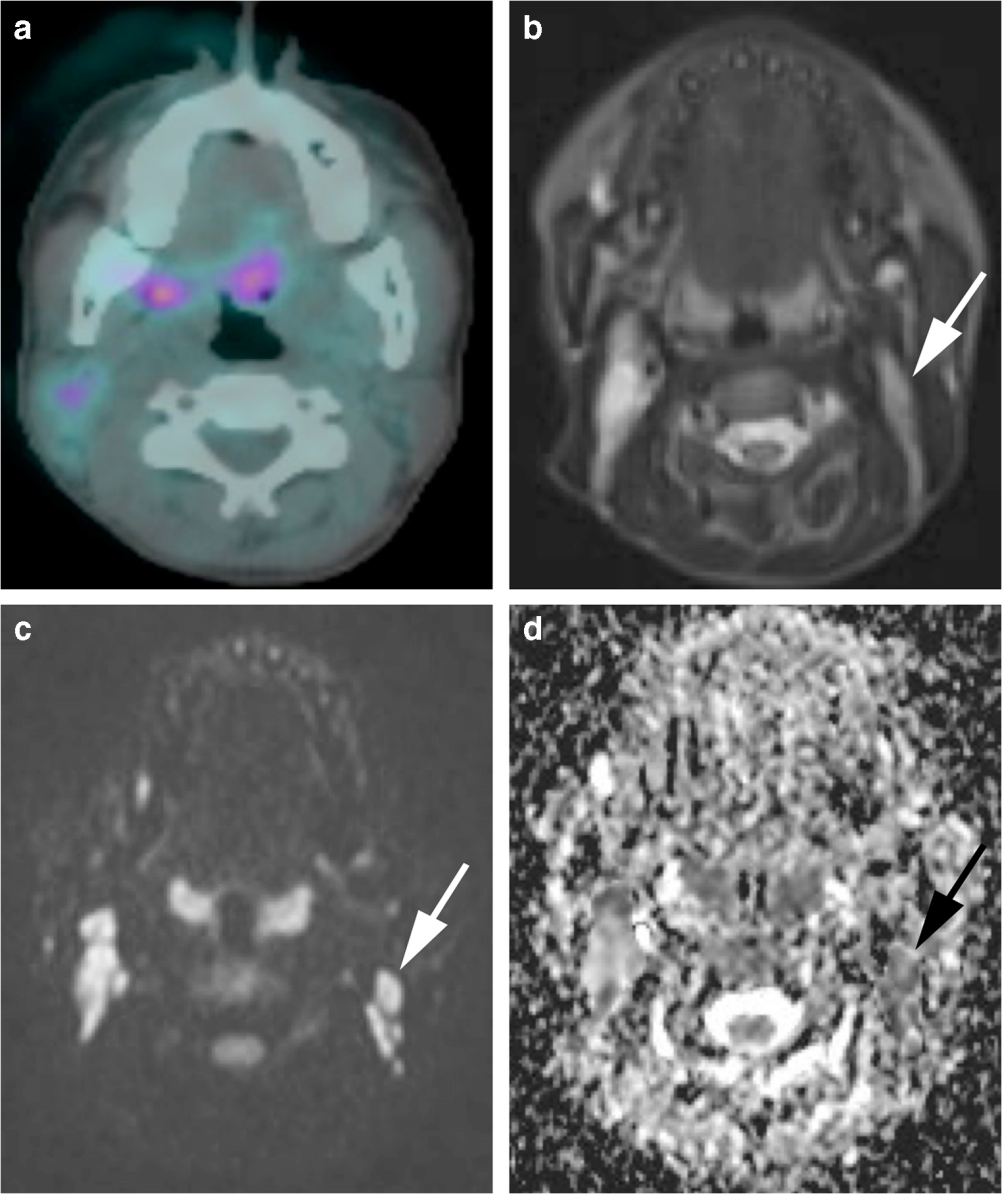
Images in a 16-year-old girl with Hodgkin lymphoma. a^18^Flourine-2-fluoro-2-deoxyglucose (FDG) positron emission tomography (PET)/CT is deemed negative for left cervical nodal involvement. b Axial short tau inversion recovery half-Fourier-acquisition single-shot turbo-spin-echo (repetition time/echo time [TR/TE] = 800/60 ms) MR image demonstrates a 5-mm short-axis-diameter left cervical node (*arrow*). c Axial diffusion-weighted imaging (DWI; b=500 s/mm^2^; TR/TE=4,900/66 ms) demonstrates a 5-mm short-axis-diameter left cervical node (*arrow*). These findings would result in true-negative nodal classification. d Apparent diffusion coefficient (ADC) map demonstrates the same left cervical node (*arrow*) with ADC of 0.9×10^−3^ mm^2^ s^−1^. The node was deemed positive on whole-body MRI by combined short-axis diameter ≥10 mm and ADC cut-off value of <1.15×10^−3^ mm^2^ s^−1^ classification — a false-positive result compared with using the FDG PET/CT reference
